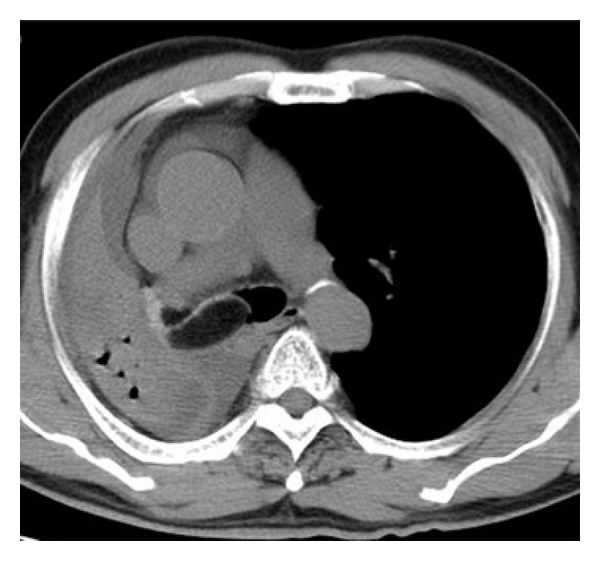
Axial CT showed hypodense mass in the right main bronchus.
